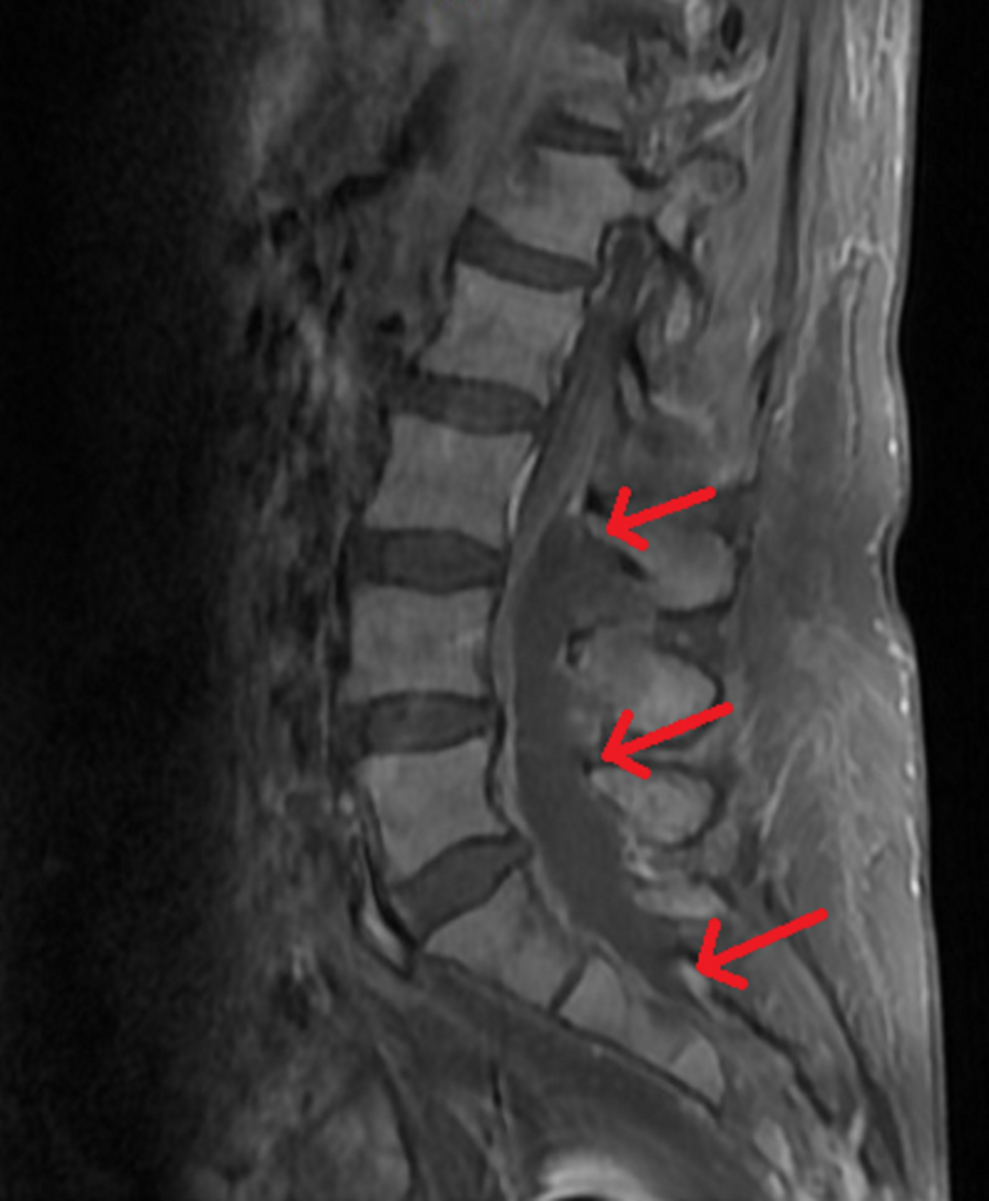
Lumbar MRI of the patient The image shows an extensive 10-cm epidural abscess from L3 to S2. Red arrows indicate the margins of the abscess MRI: magnetic resonance imaging
